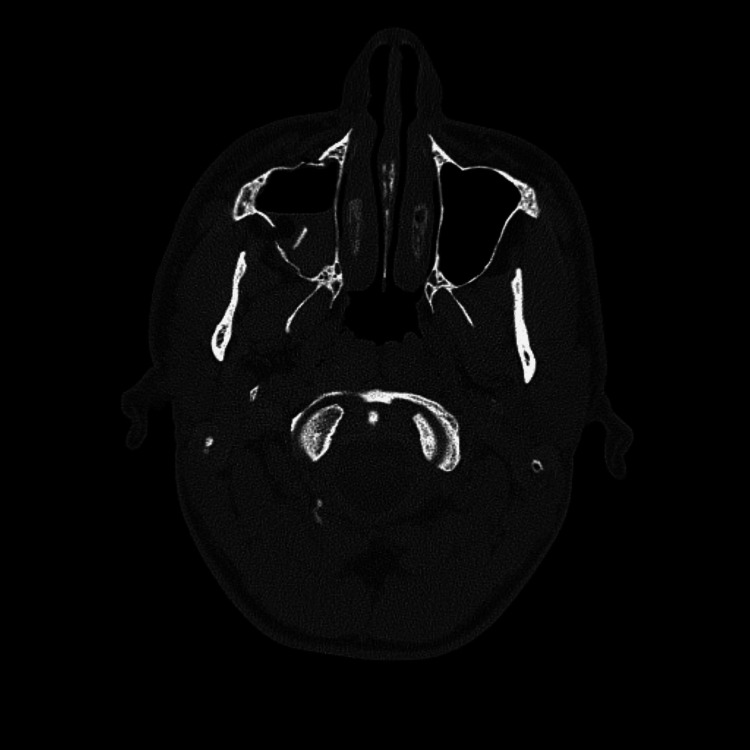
Cranial computed tomography of the patient after the trauma Transverse section of the cranial computed tomography of the patient after the trauma indicated a displaced fracture at the right posterolateral wall of the maxilla and a resulting hematoma in the maxillary sinus.
